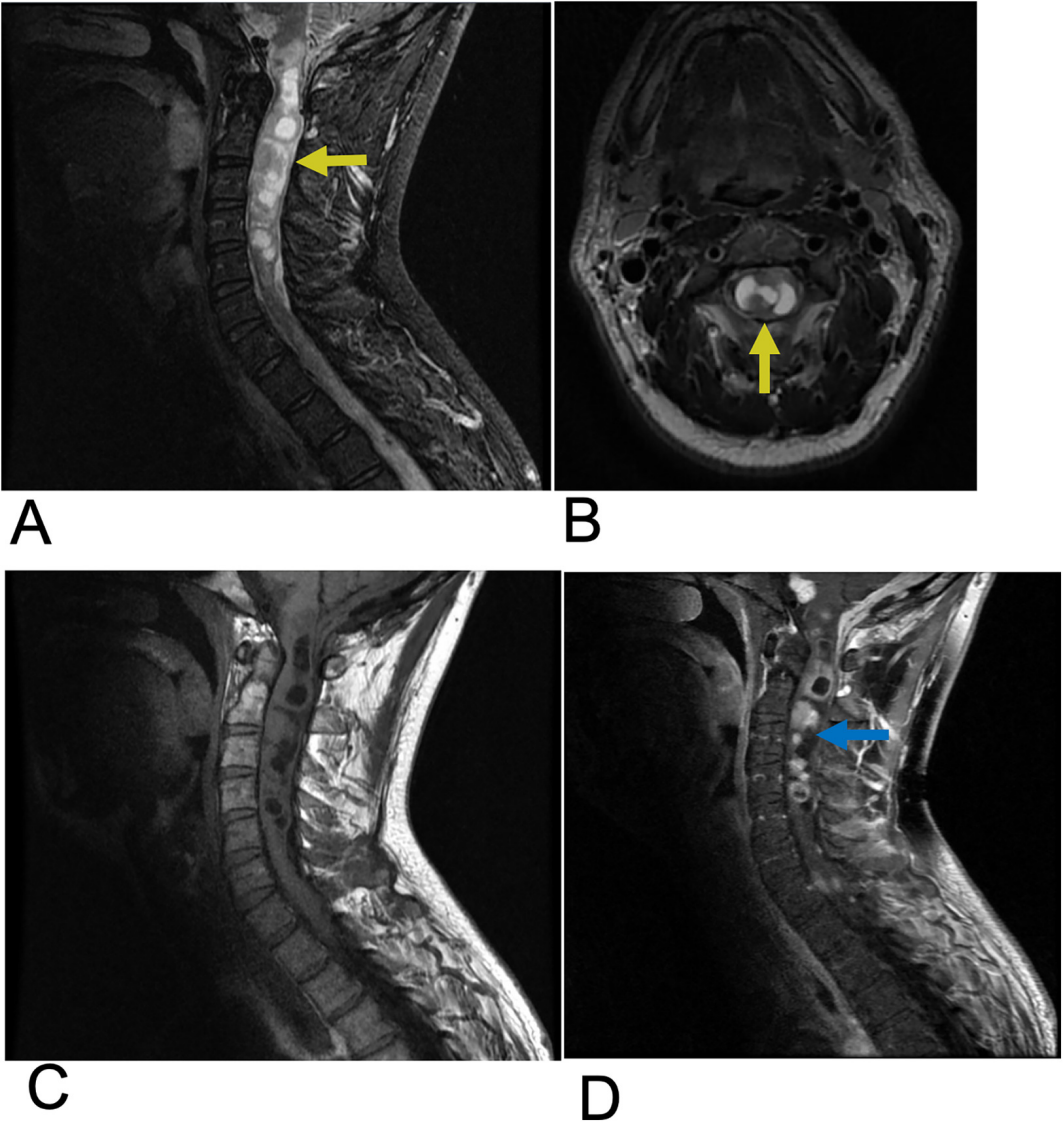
Ependymoma. Sagittal T2 (A), Axial T2 (B), Sagittal T1 FLAIR (C), and sagittal T1 FLAIR FAT + C (D) MRI of the cervical spine demonstrating intramedullary expansile multiloculated T2 hyperintense cystic lesions (yellow arrows) in the cervical cord spanning from C2–C5 with edema at the inferior aspect of the lesions. There are associated enhancing tumoral nodules in the spinal cord representing spinal ependymoma (blue arrow). No evidence of hemorrhage.
